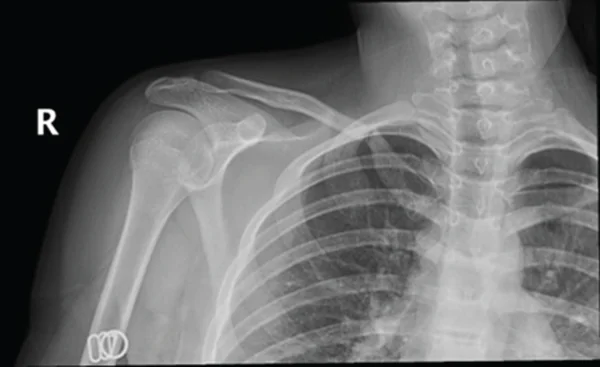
Day 0 radiographs demonstrating a proximally angulated right medial clavicular shaft fracture obtained in the emergency department.
